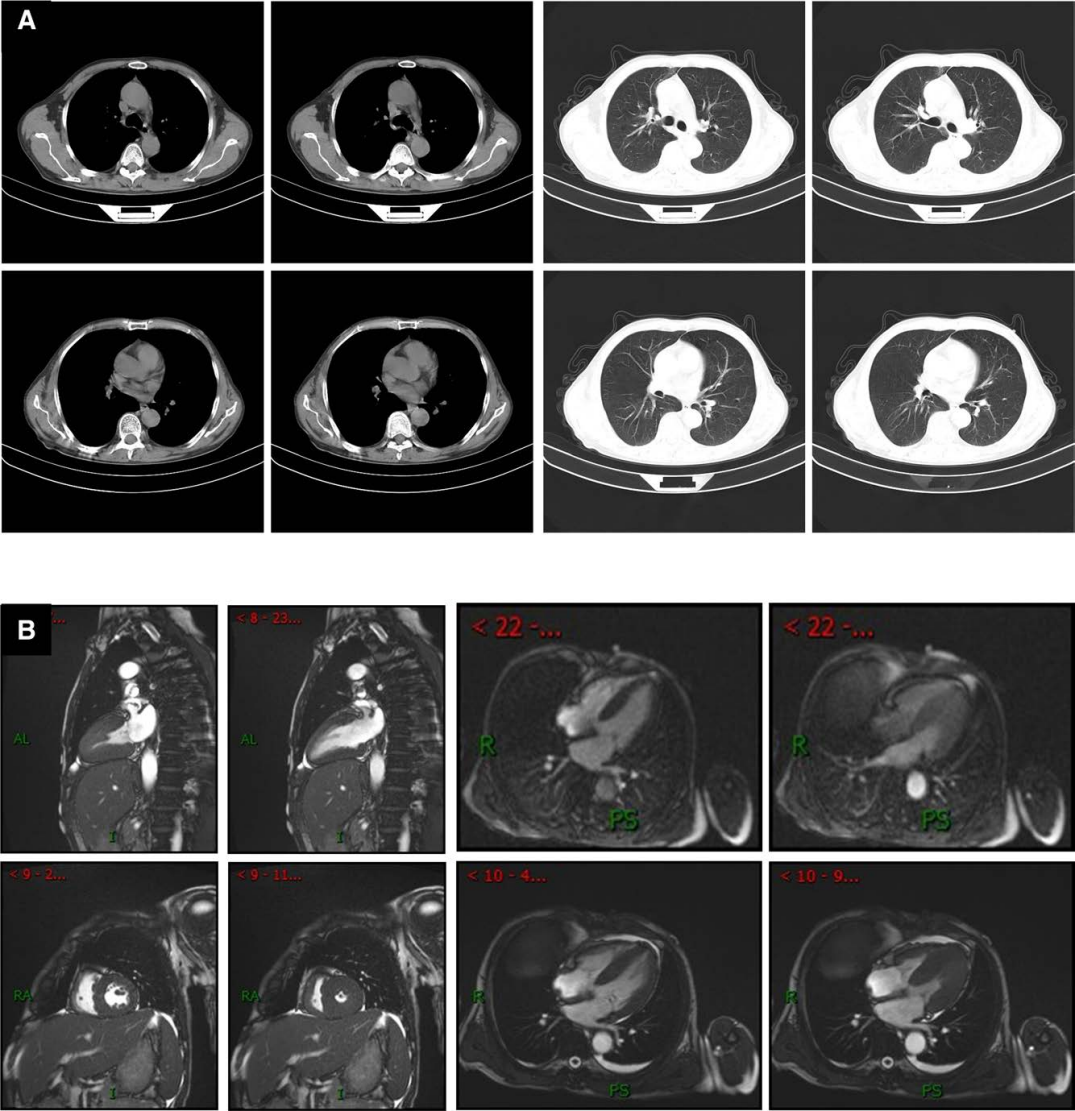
Computed tomography (CT) and magnetic resonance imaging (MRI). (A) Chest CT (November 18, 2021) shows slight streaks and patchy shadows with increased density in both lungs. The trachea and bronchi of each lobe are unobstructed, and the mediastinum is centered. No lymph nodes are found in the mediastinum or hilum. Left ventricular enlargement, and enlargement and decreased density of the liver are seen. (B) Plain and enhanced cardiac MRI scans (February 22, 2022) show uniform thickening of various segments of the left ventricular wall, and linear enhancement shadows around the subendocardial layer of the proximal left ventricle, right atrium, and right ventricular inflow tract, which is consistent with the MRI manifestations of myocardial amyloidosis. A small amount of pericardial effusion, and mild mitral and tricuspid regurgitation are detected. Left ventricular myocardial compliance is decreased, and diastolic function is slightly weakened. Minor pleural effusion is present on both sides.
